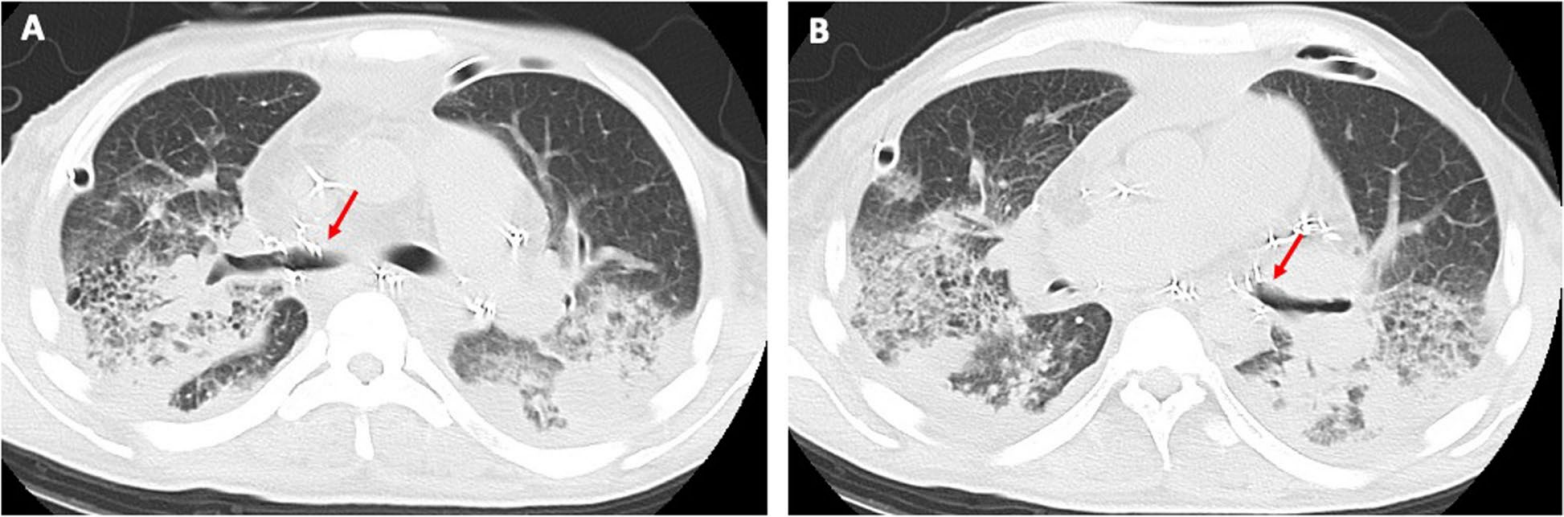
A, B The follow-up CT scan 12 days after the surgical repair revealing good healing of the bilateral dehiscence (red arrows)
